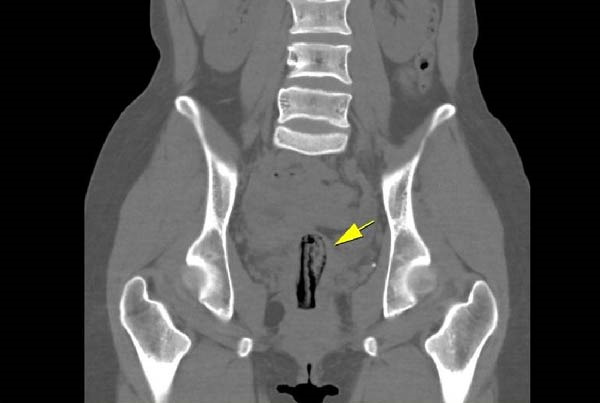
Coronal abdominal CT scan showing a linear high‐density (hyperdense) structure centrally located within the uterine cavity (yellow arrow), subsequently confirmed via pelvic examination to be an intrauterine tampon.
